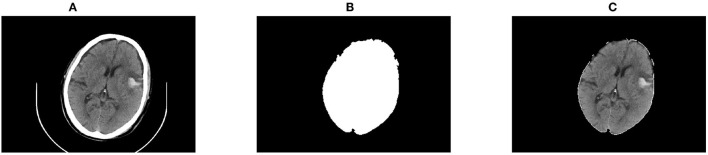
Results of the first region growing. (A) The original CT image; (B) Segmentation of the first region growing; (C) Restoration of the first region growing results.
